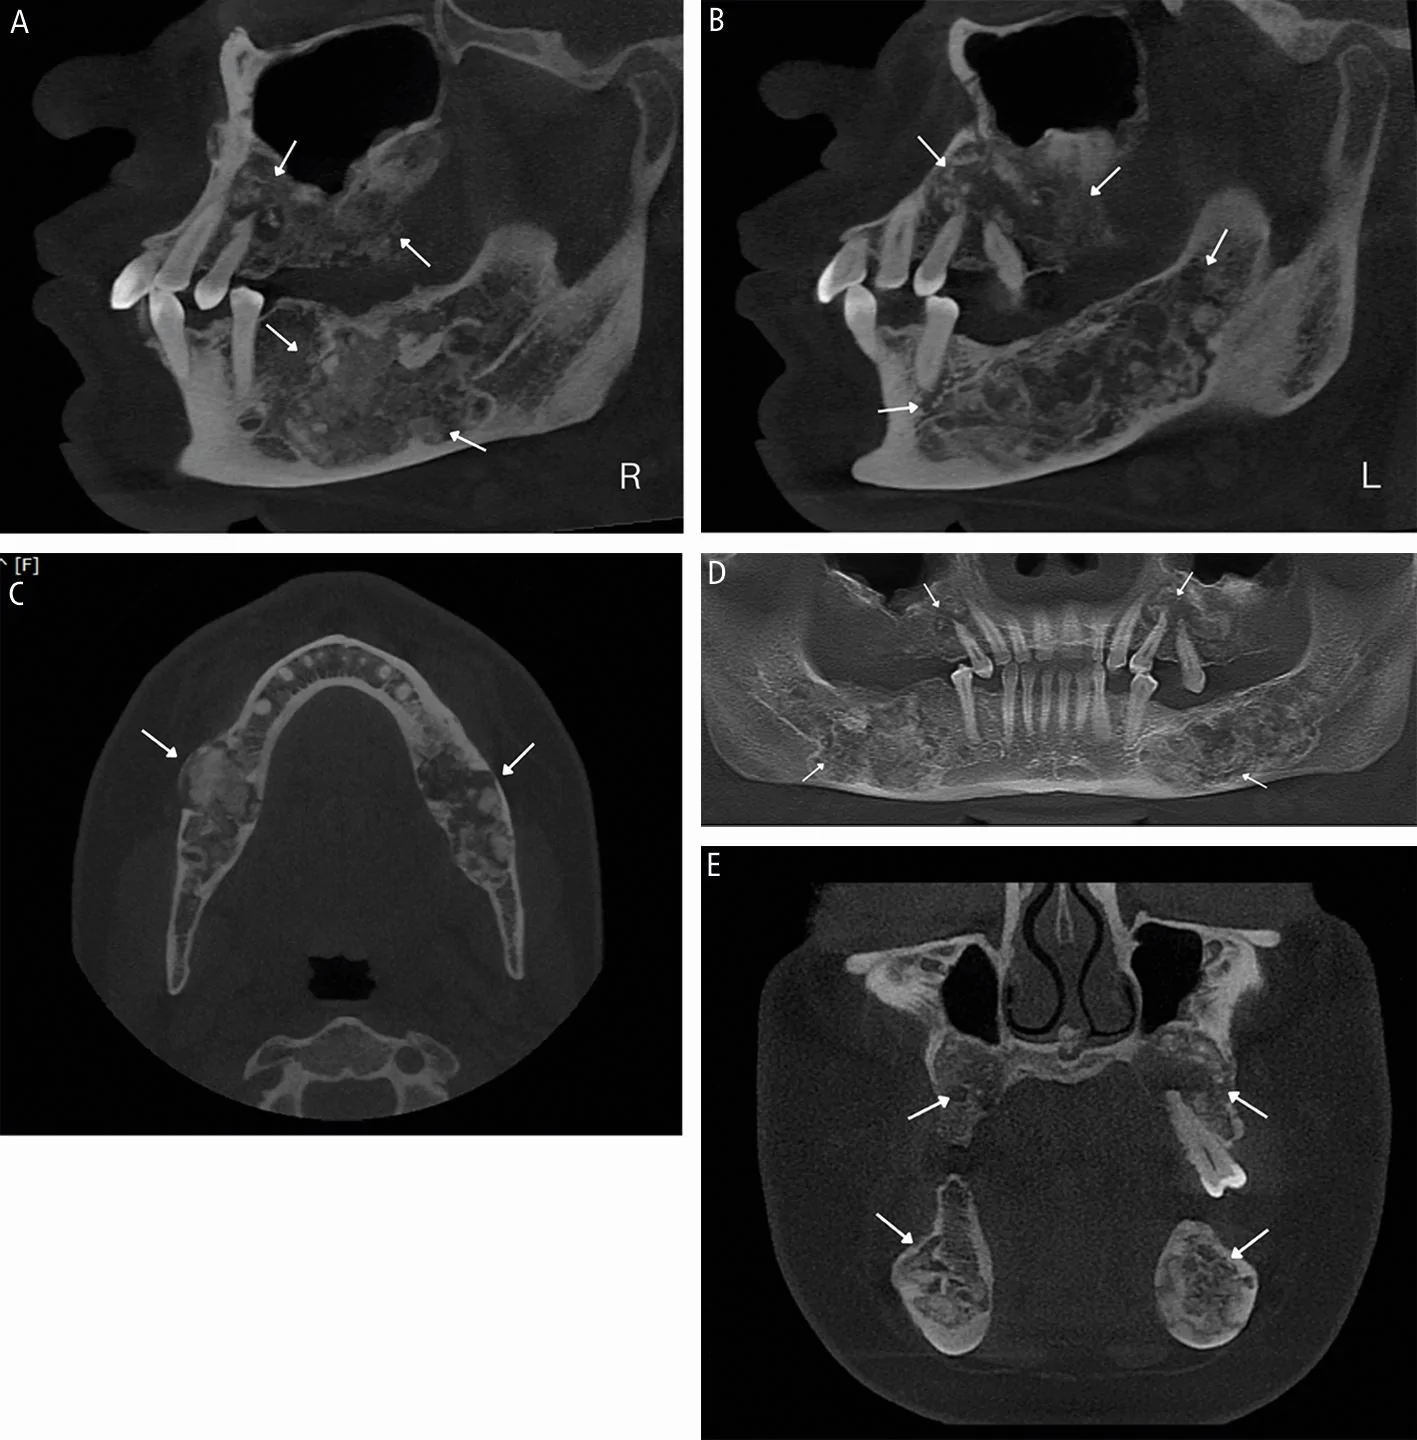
Sagittal (A, B), axial (C), panoramic (D), and coronal (E) CBCT reconstructions of Case 1 at 29 years of age, demonstrating mixed-density lesions in all quadrants (arrows), with buccolingual bone expansion in both the maxilla and mandible
